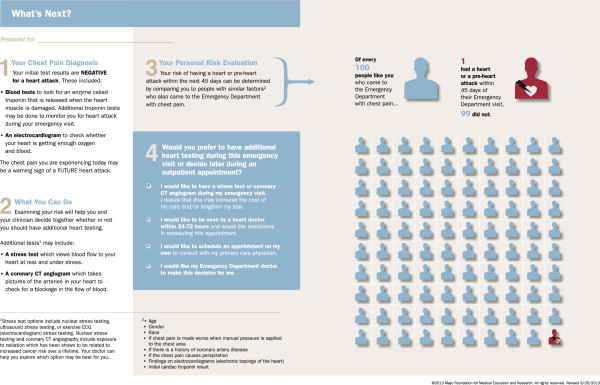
Decision aid that includes the option of coronary CT angiography. This version of the decision aid was developed for use at the University of Pennsylvania emergency department (Pennsylvania, United States) in which coronary CT angiography is frequently used in lieu of cardiac stress testing.
